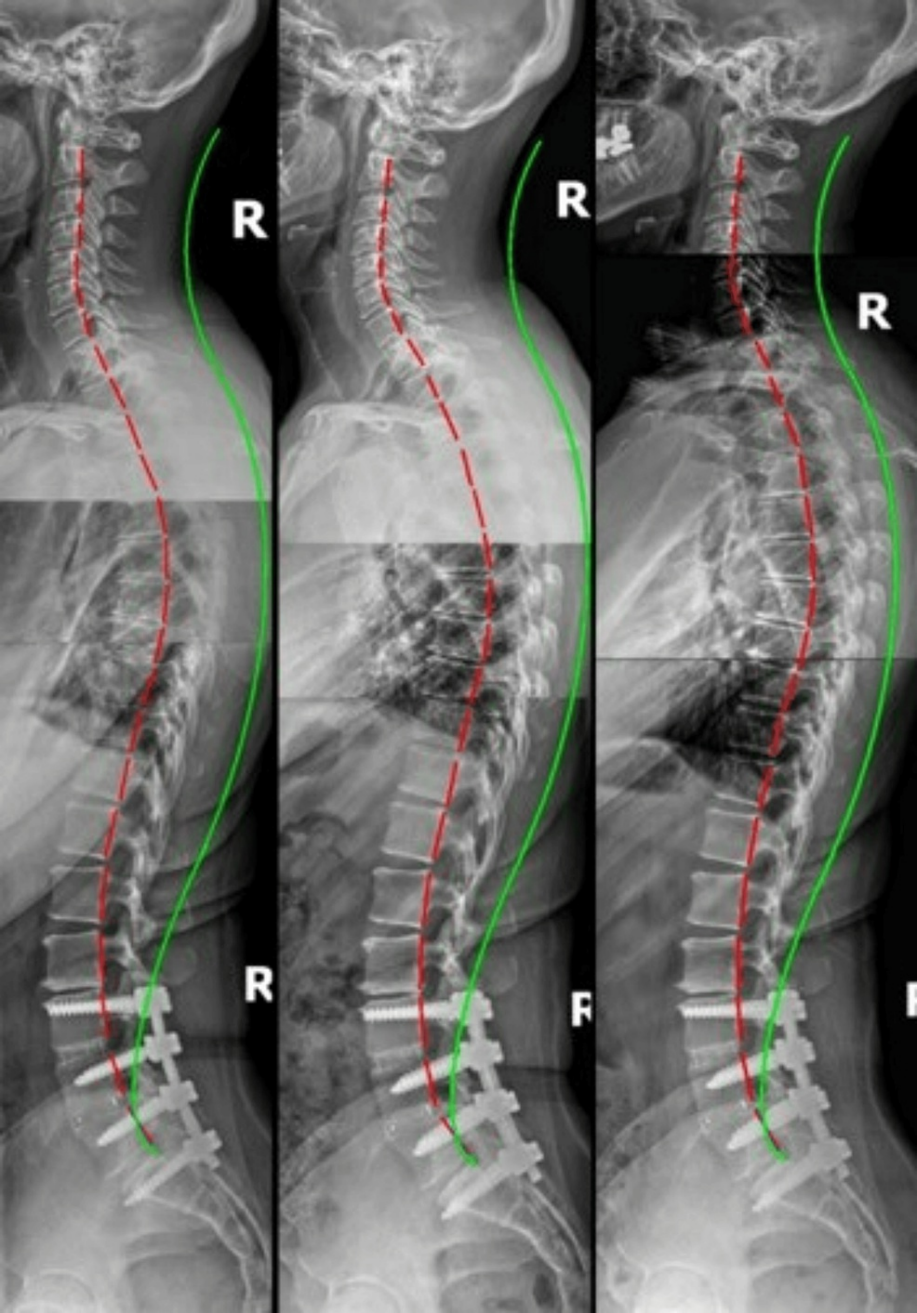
Lateral spinal radiographs Left: Initial; Middle: Post-treatment; Right: Six-month follow-up. Green lines indicate ideal alignment; red lines highlight the patient’s spinal position.
